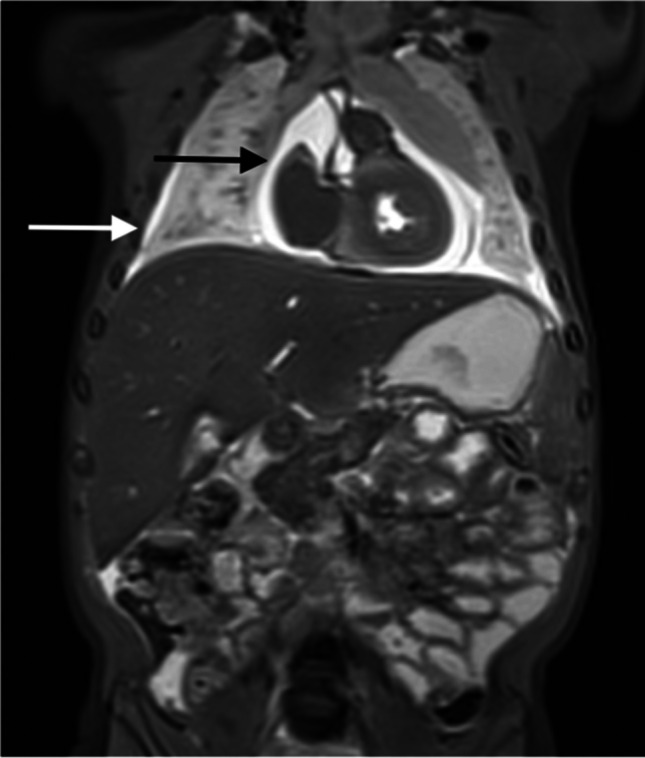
Normal thoracoabdominal postmortem magnetic resonance imaging in a 3-month-old boy. Coronal T2-weighted image shows a small amount of pericardial fluid (*black arrow*) and pleural fluid (*white arrow*), consistent with normal postmortem changes
